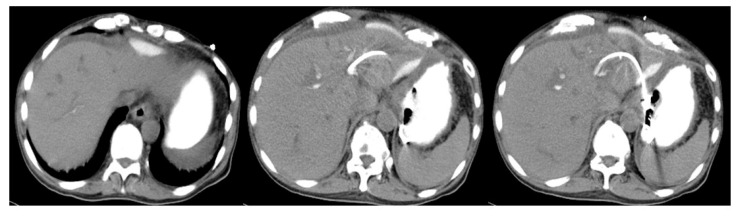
CT scan image showing bile leak. Contrast medium is filling the stomach. Leakage of contrast medium can be seen near the stenting site.
